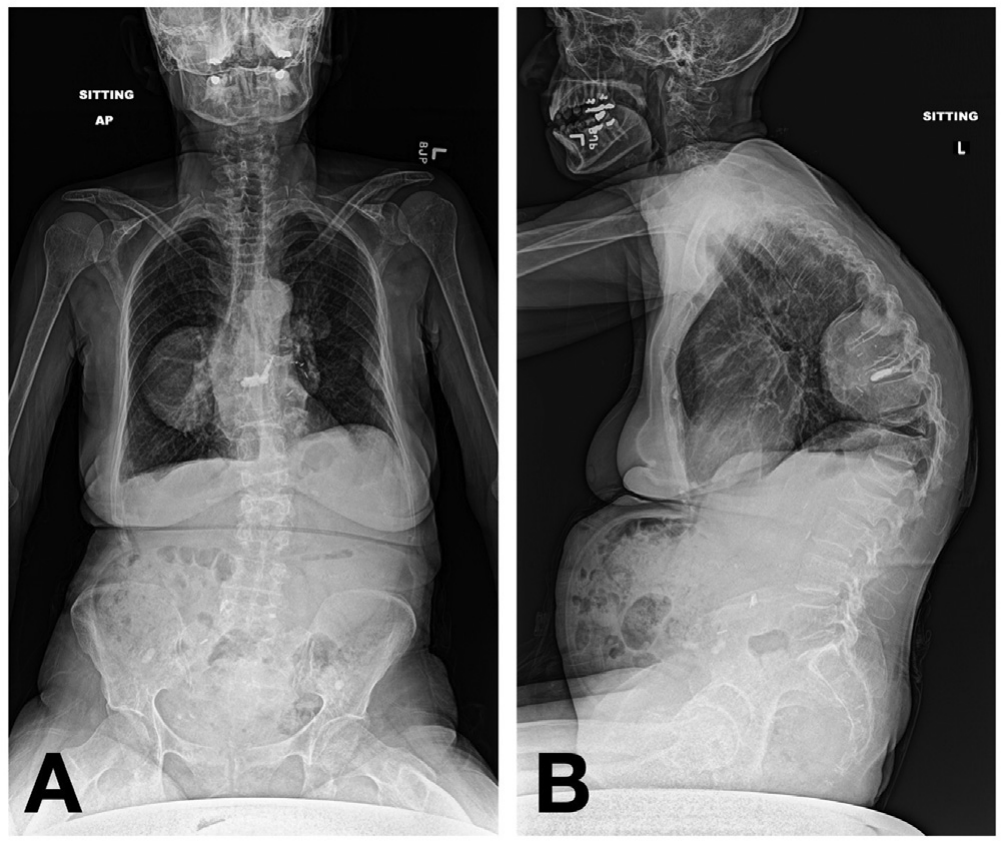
AP (A) and lateral (B) scoliosis x-rays at 3-week postoperative visit demonstrating maintained alignment of the spinal column with no cement extravasation or new loss of height.
